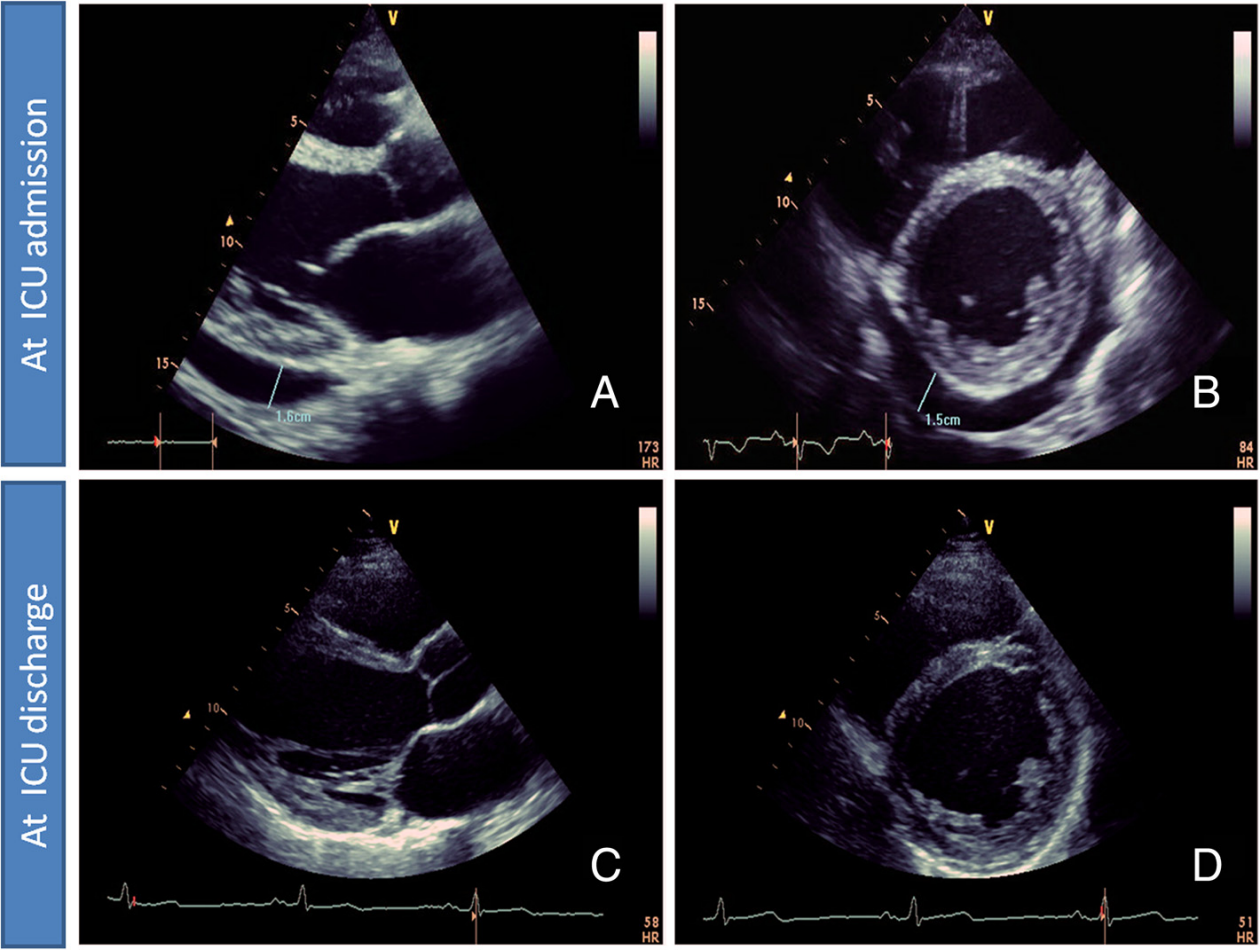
Transthoracic cardiac echocardiogramms. At ICU admission: (A) long axis view, and (B) short axis view; both views show a pericardial effusion of 1.6 cm. At ICU discharge: (C) long axis view, and (D) short axis view; both views confirm significant reduction of pericardial effusion.
